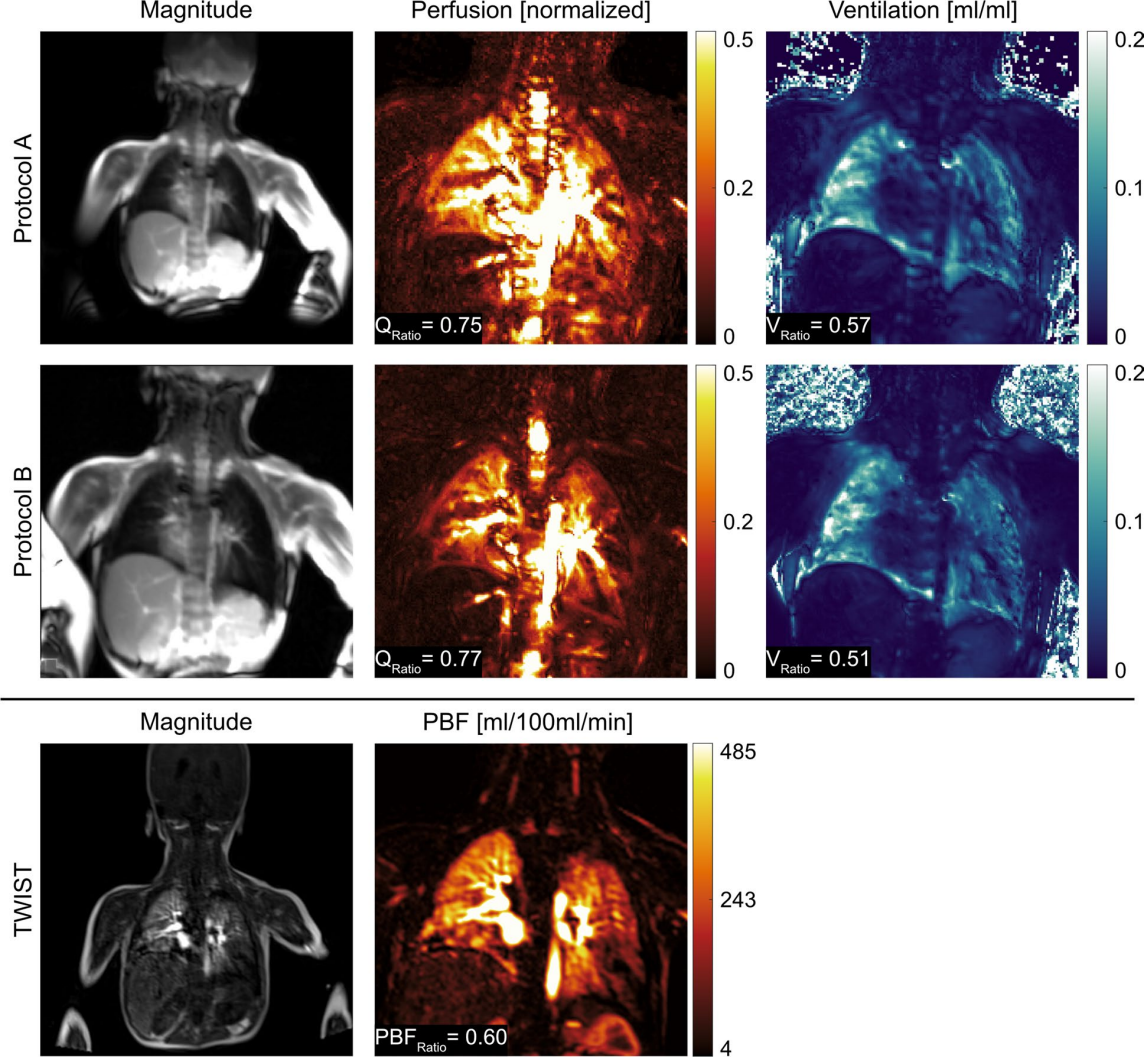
Representative magnitude images from bSSFP protocols, along with normalised perfusion, and fractional ventilation maps obtained through DMD MRI from a patient with left-sided hernia and ECMO therapy (patient no. 5). Additionally, a representative magnitude image and PBF map obtained from DCE-based TWIST protocol for the same patient from a similar slice location are displayed. For visualisation purposes, all functional maps are cropped to the region of interest. Overall, reductions in the ipsilateral lung can be observed visually, and can be confirmed by the resulting quantitative ratios (displayed overlaid on functional maps). Although the TWIST-based method can generate quantitative PBF maps, DMD MRI does not require the administration of contrast agents and is also capable of generating ventilation maps
